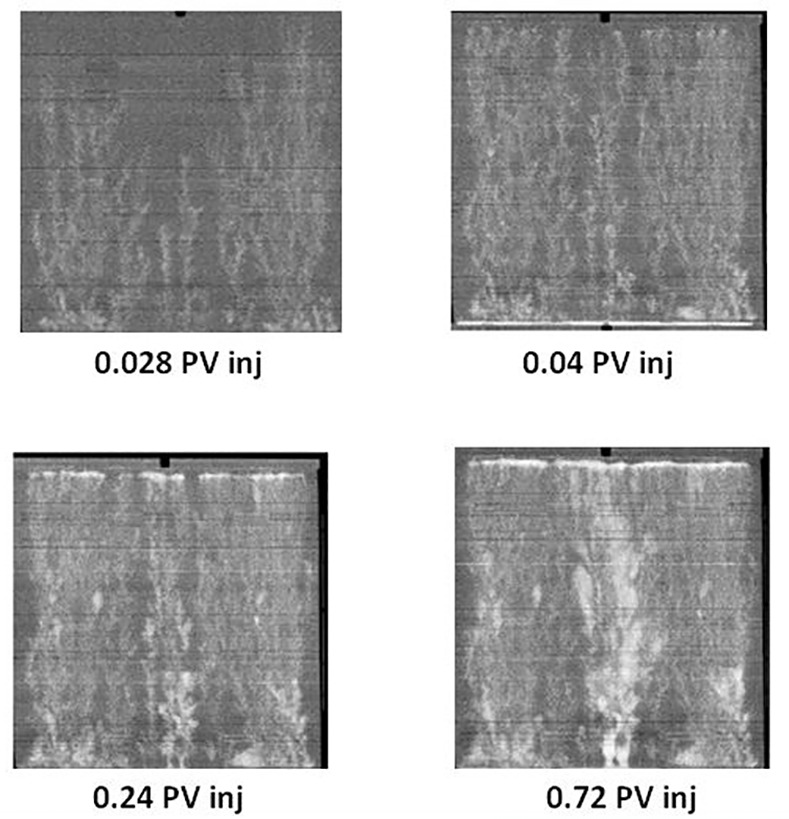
X-Ray images of saturation maps of E7000 experiment for different injected pore volumes [2]. The water (white) is injected from the bottom to displace the oil (black). Note that a pore volume (PV) is a unit used to report the cumulative volume of water injected since the start of a displacement: e.g. 1PV means that the cumulative volume of water injected so far is equal to the total volume of the pore space.
